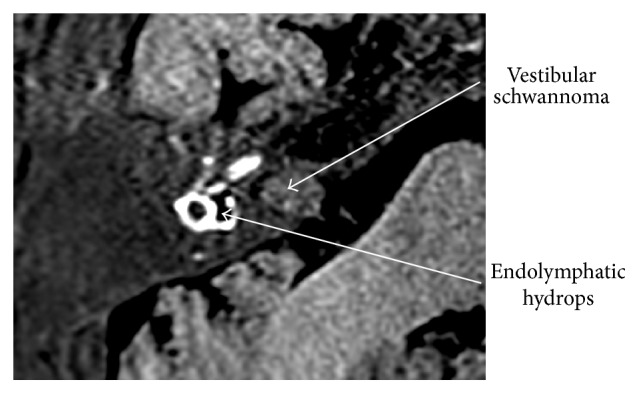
Endolymphatic hydrops and VS seen in a “locally enhanced inner ear MR imaging” (LEIM), 3D real inversion recovery MRI of the right inner ear after intratympanic Gadolinium contrast application. The vestibulum, the horizontal SCC and the basal turn of the cochlea are visible. Perilymph appears hyperintense, endolymph appears hypointense (black), and surrounding temporal bone appears grey. The vestibular endolymphatic space is clearly enlarged, indicating endolymphatic hydrops.
